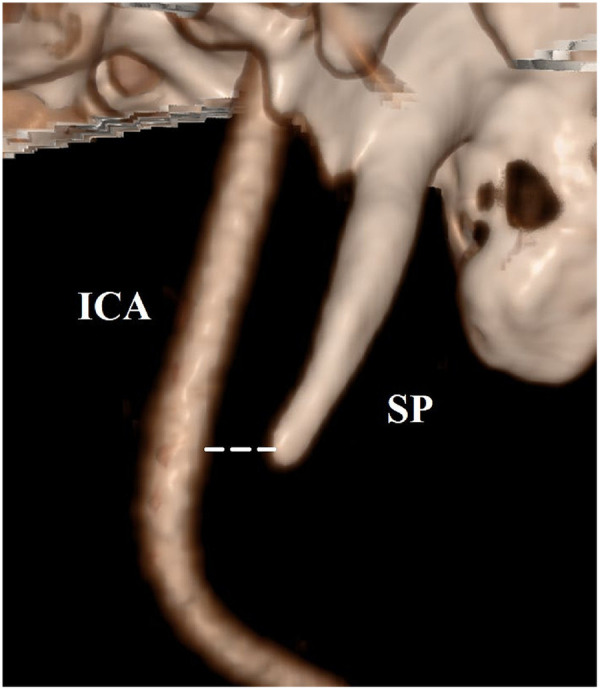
Measurement of the marginal shortest SP-ICA distance using axial view on 3D reconstruction of the CTA (the shortest marginal SP-ICA distance is marked with white dotted lines). CTA, computed tomography angiography; ICA, internal carotid artery; SP, styloid process.
